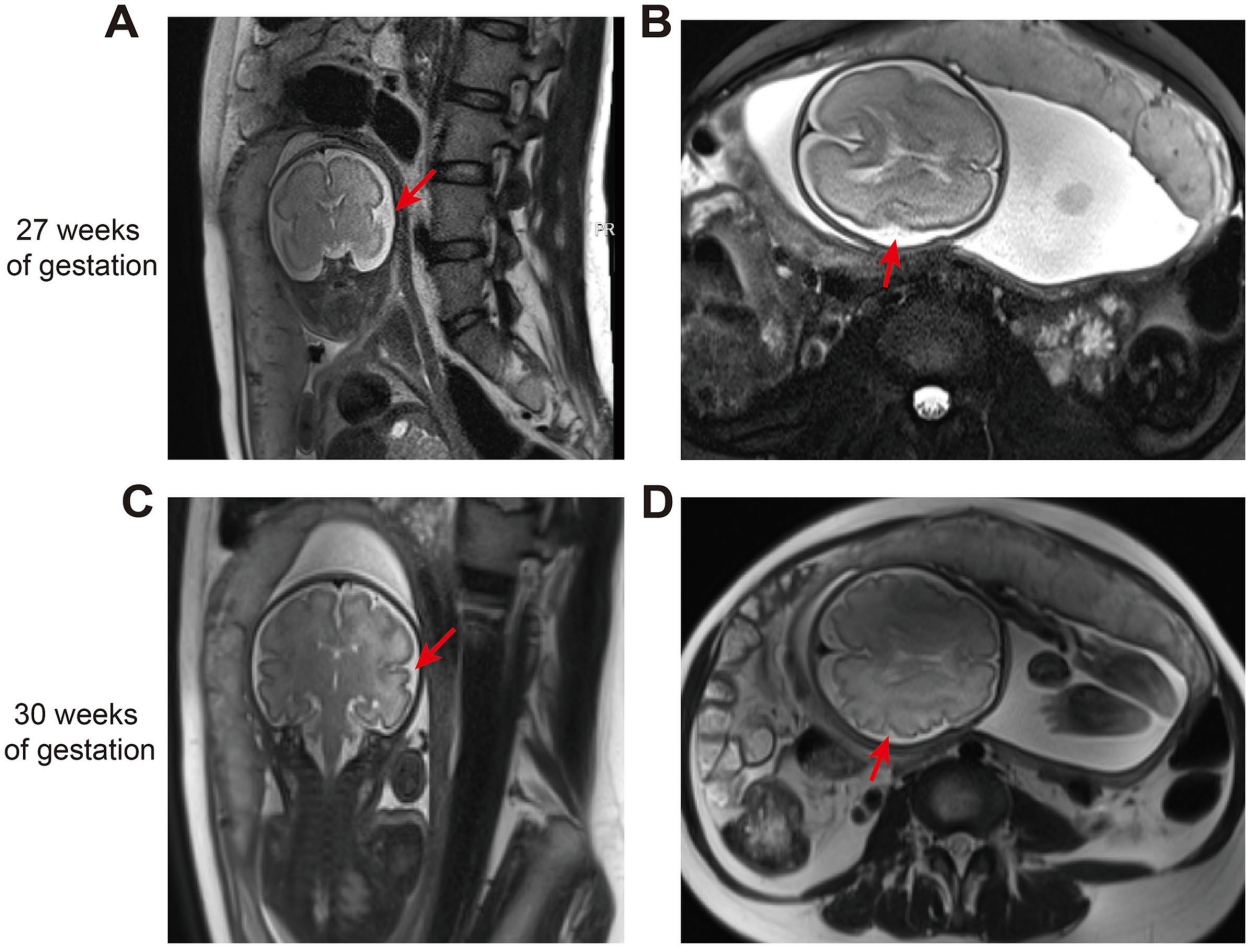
The fetal MRI findings. (A,B) The fetal brain MRI showed focal cortical thickening and sulcal deepening at the left temporoparietal junction at 27 weeks of gestation. (C,D) The fetal brain MRI showed progressive sulcal deepening and cortical thickening at the left temporoparietal region, with adjacent white matter hyperintensity.
